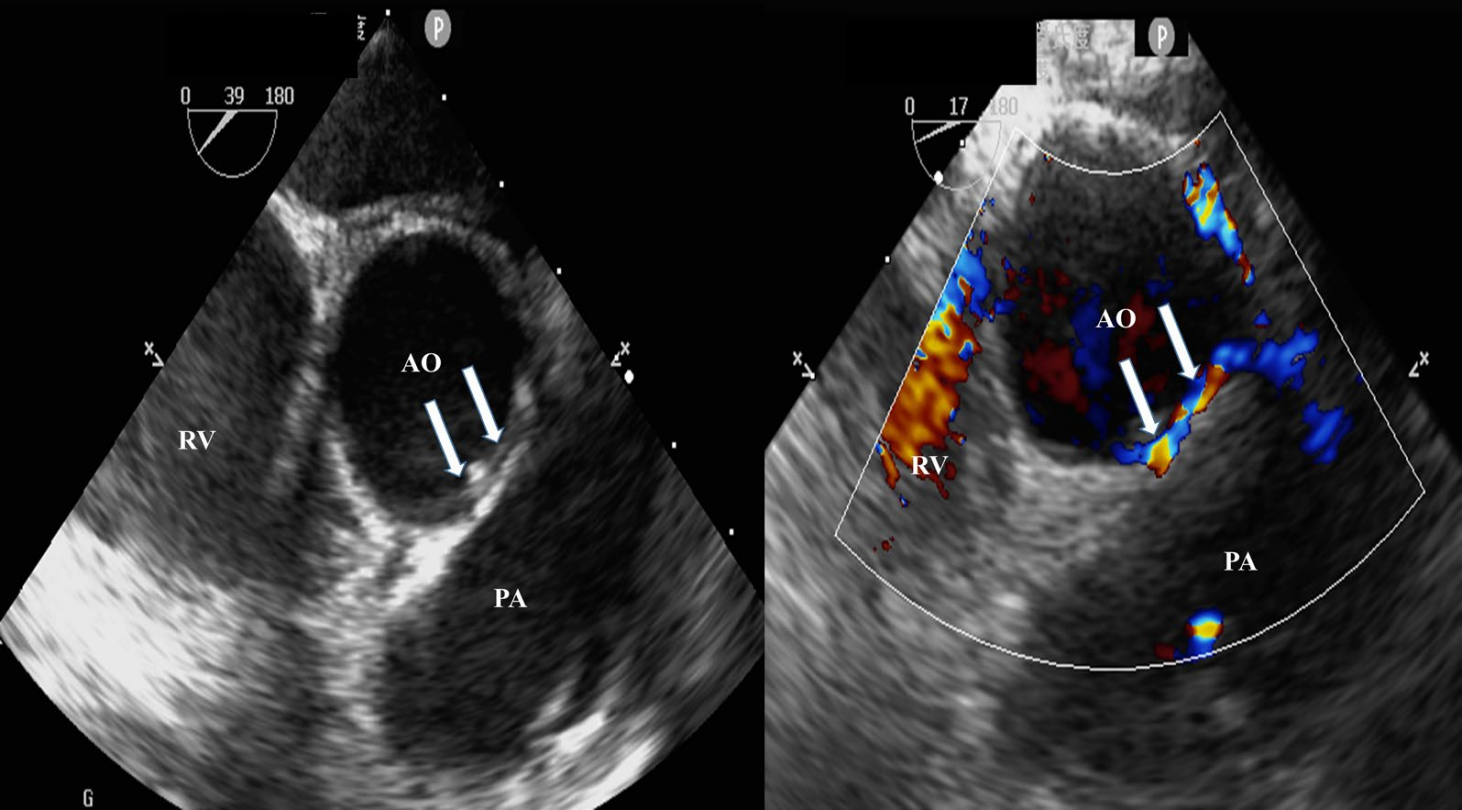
Transoesophageal echocardiography revealed the anomalous LCA and RCA arising more posteriorly and leftward from the right sinus of Valsalva and coursing intramural within the anterior aortic wall (arrows) between the Ao and PA. *LCA* left coronary artery, *RCA* right coronary artery, *Ao* aorta, *PA* pulmonary artery
